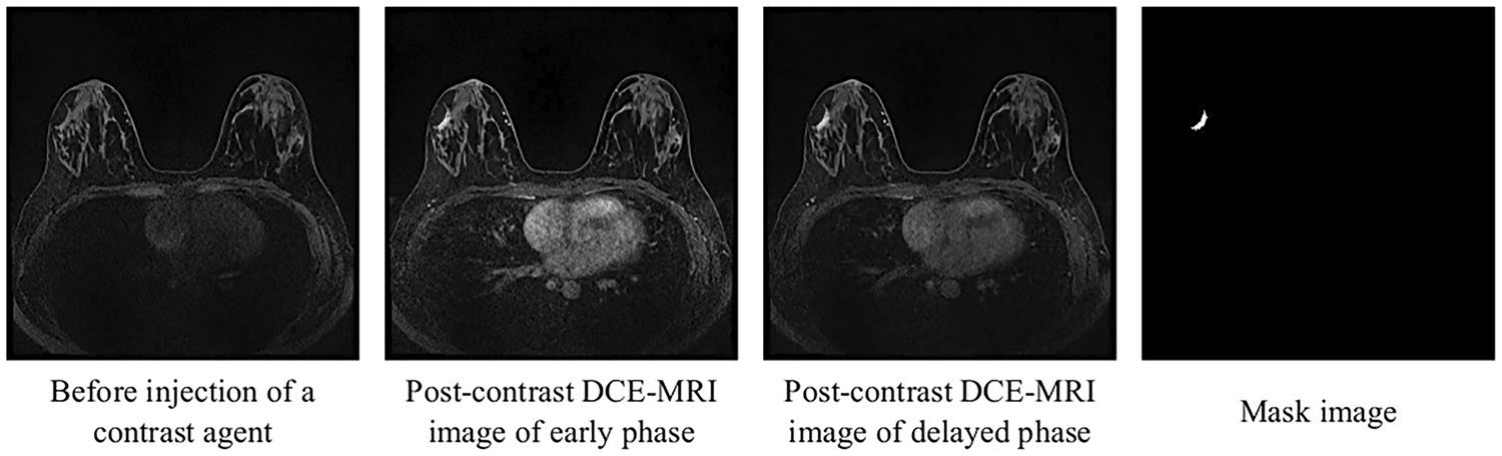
Example of pre-contrast DCE-MRI image, post-contrast DCE-MRI images, and mask image
